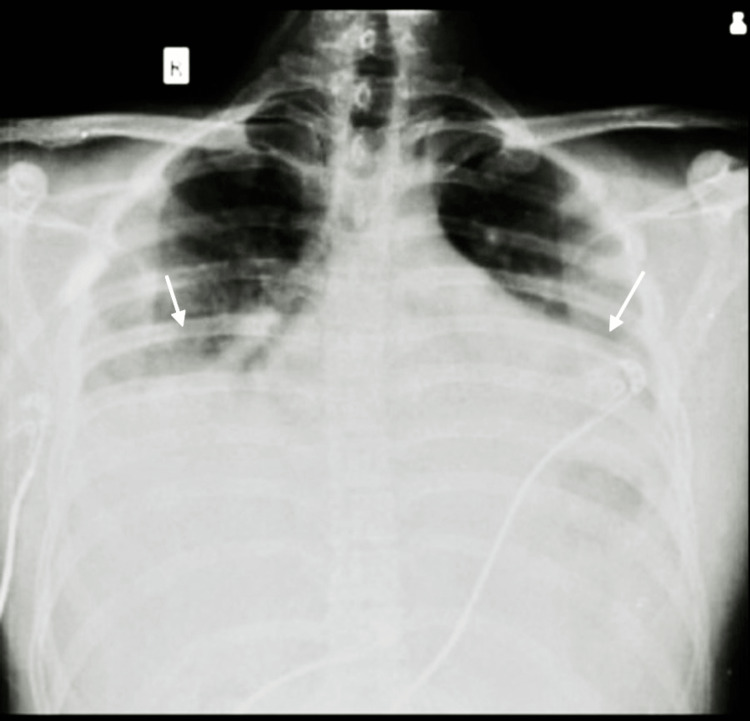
Chest X-ray showing bilateral opacities (arrows)
